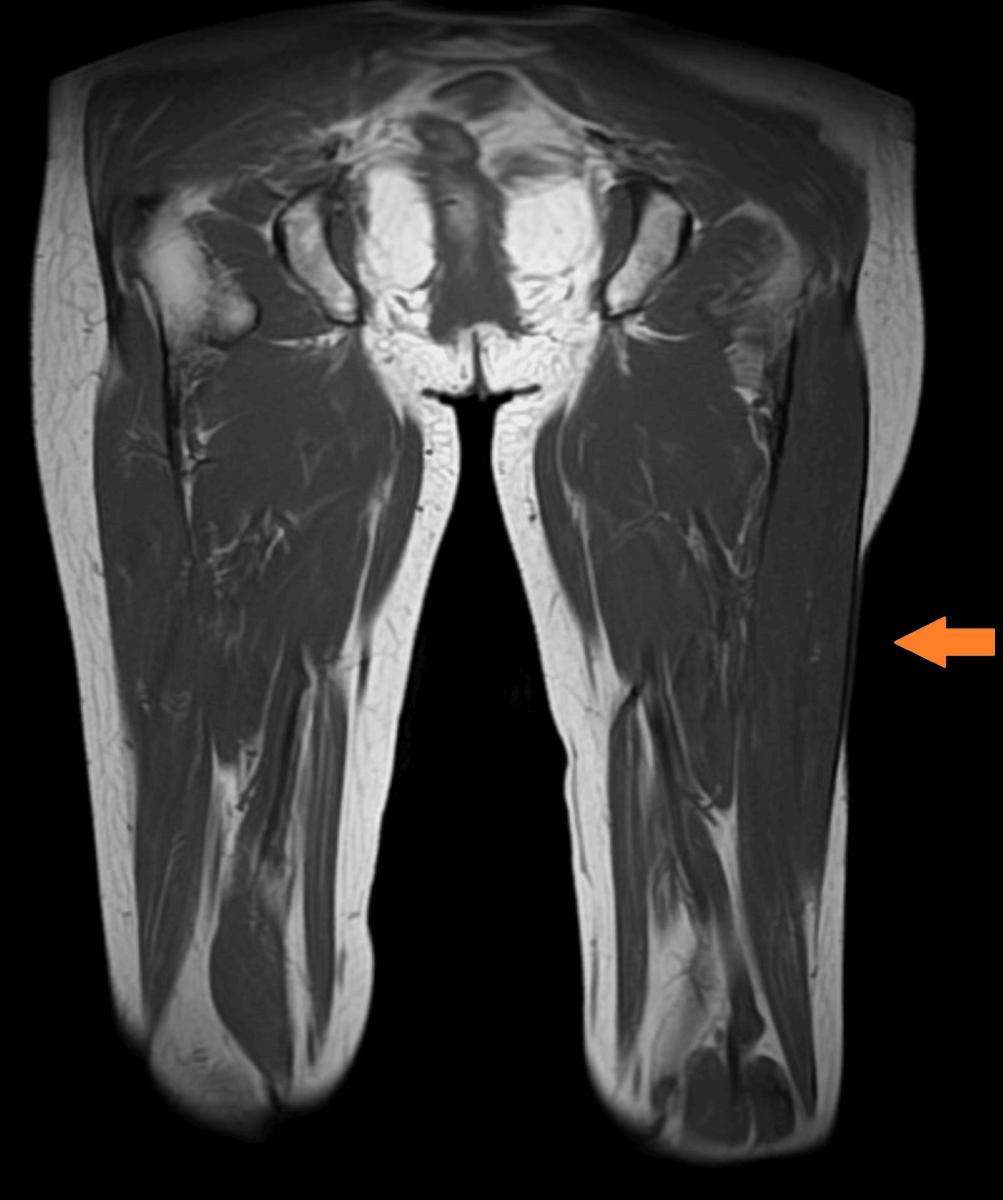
T1-weighted images on MRI of the thigh The figure shows skin depression on the left thigh. No muscle atrophy was observed, but there was a noted loss of approximately 8 cm of subcutaneous adipose.
